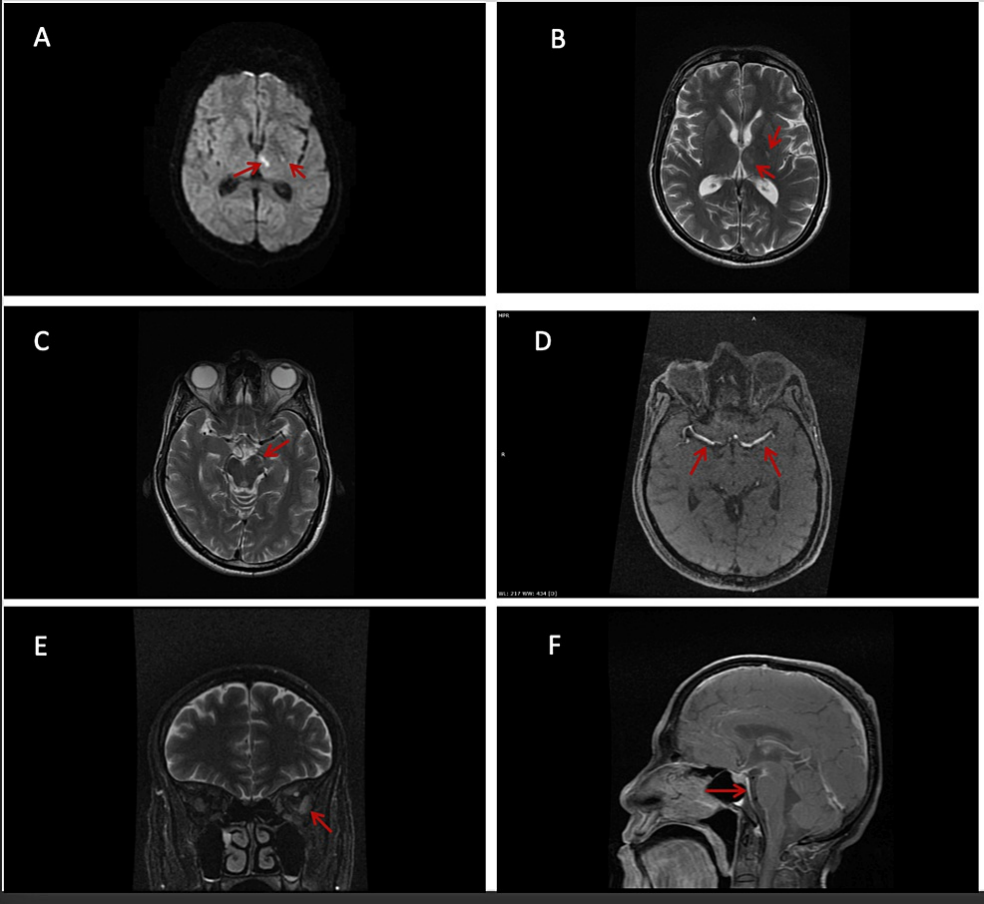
MRI of the Orbit and Brain With Angiography (A) T2 FLAIR image showing hyperintensity in the left thalamus and capsuloganglionic region (red arrows). (B) T2-weighted image showing hyperintensity in the left thalamus and capsuloganglionic region (red arrows). (C) T2-weighted image showing subtle hyperintensity in the left cerebellar peduncle of the midbrain (red arrow). (D) T1-weighted post-gadolinium axial image showing thickening and enhancement of the walls of the bilateral middle cerebral arteries (red arrows). (E) T2-weighted coronal image showing bulky left lateral rectus muscle (red arrow). (F) T1 post-gadolinium sagittal image showing abnormal prepontine leptomeningeal enhancement (red arrow).
